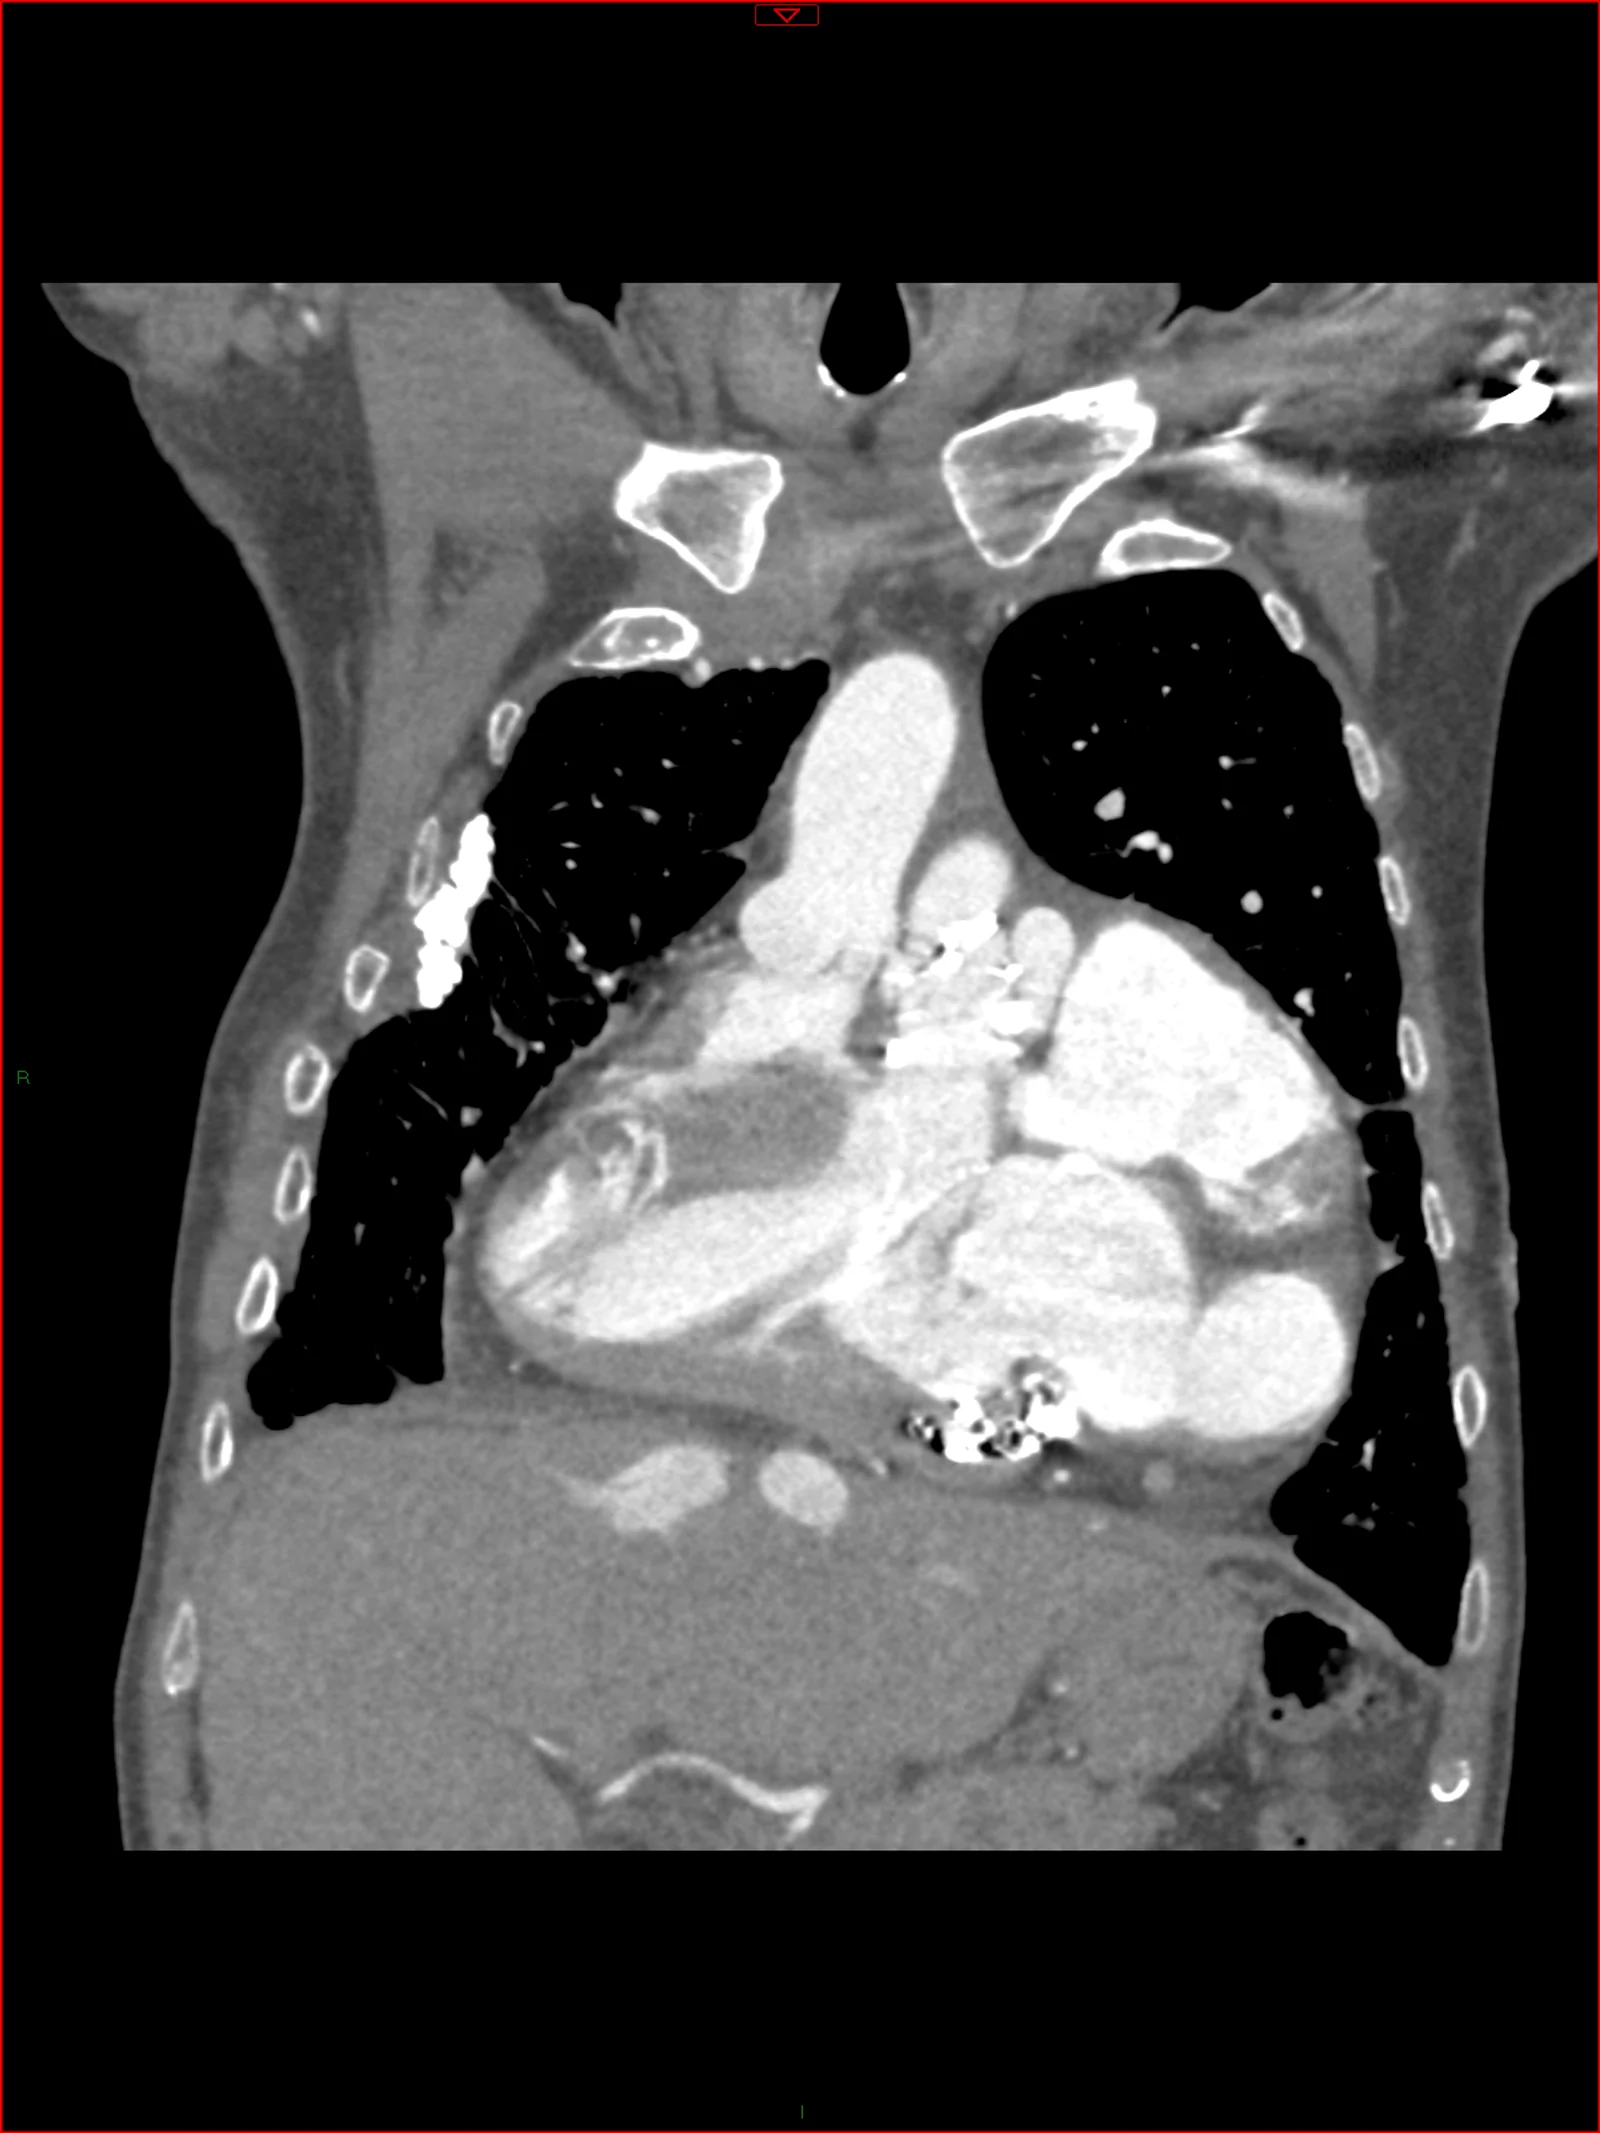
Chest CT angiography with coronal reconstruction demonstrating mesocardia and cardiomegaly CT: computed tomography
